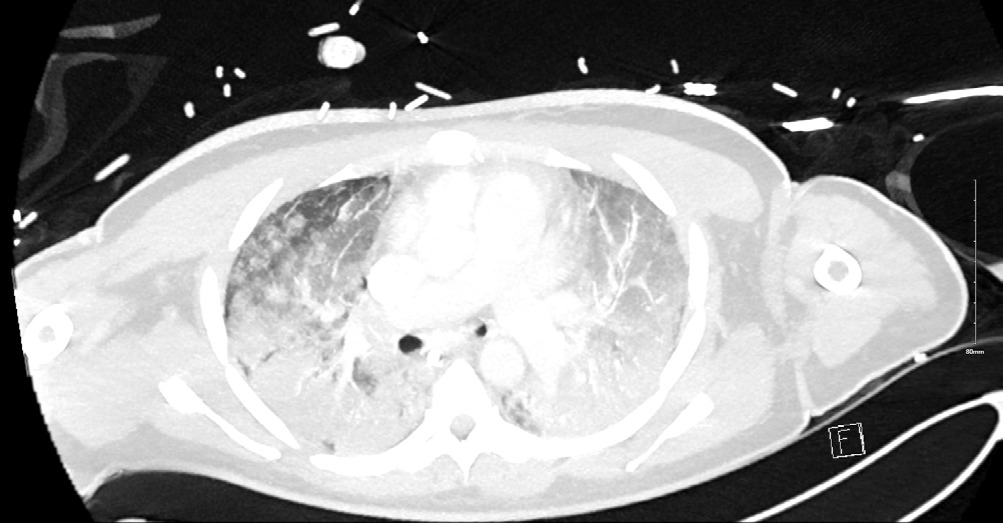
CT Thorax on Day 15 after clinical deterioration showing dense bilateral infiltrates throughout both lung in keeping with ARDS and possible organizing pneumonia. There was no pneumothorax or pleural effusions.
